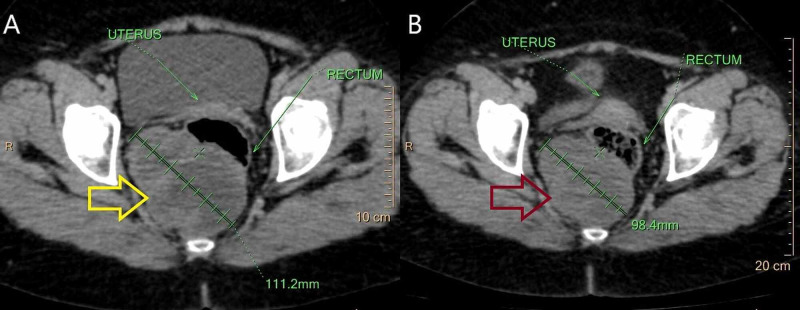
(A) Pelvic CT scan showing a large tumor of the posterior rectal wall (yellow arrow). (B) Repeated pelvic CT scan after one year of neoadjuvant Imatinib therapy showing a reduction in the tumor size of 18.2% (red arrow)
